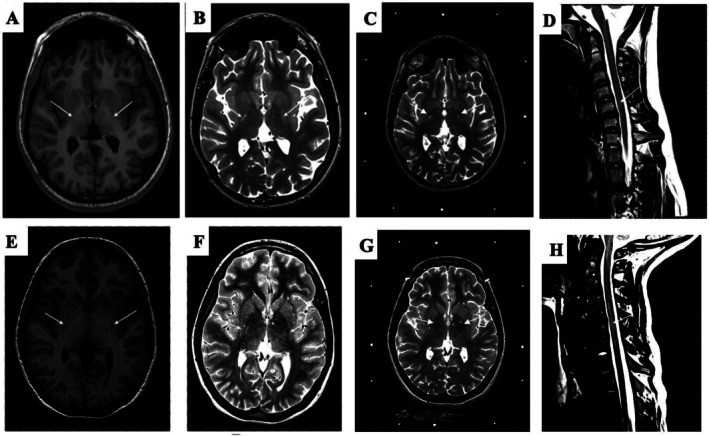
Case 1: (A) T1‐weighted images show bilateral motor putamen atrophy (white arrows), producing an “Olympic torch” appearance with a radish‐tail configuration, typically seen in perinatal anoxic brain lesions. (B) Corresponding T2‐weighted images demonstrate bilateral putaminal hyperintensities (white arrows). (C) Postoperative T2‐weighted images confirm bilateral DBS (deep brain stimulation) lead implantation in the GPi (internal globus pallidus, white arrow). (D) Cervical spinal MRI (magnetic resonance imaging) reveals centro‐medullary T2 hyperintensities (white arrow). Case 2: (E) T1‐weighted images show mild bilateral motor putamen atrophy (white arrows). (F) T2‐weighted images demonstrate bilateral motor putamen hyperintensities (white arrows). (G) Postoperative T2‐weighted images confirm bilateral DBS lead implantation in the GPi (white arrow). (H) Cervical spinal MRI reveals centro‐medullary hyperintensities on T2‐weighted images (white arrows).
